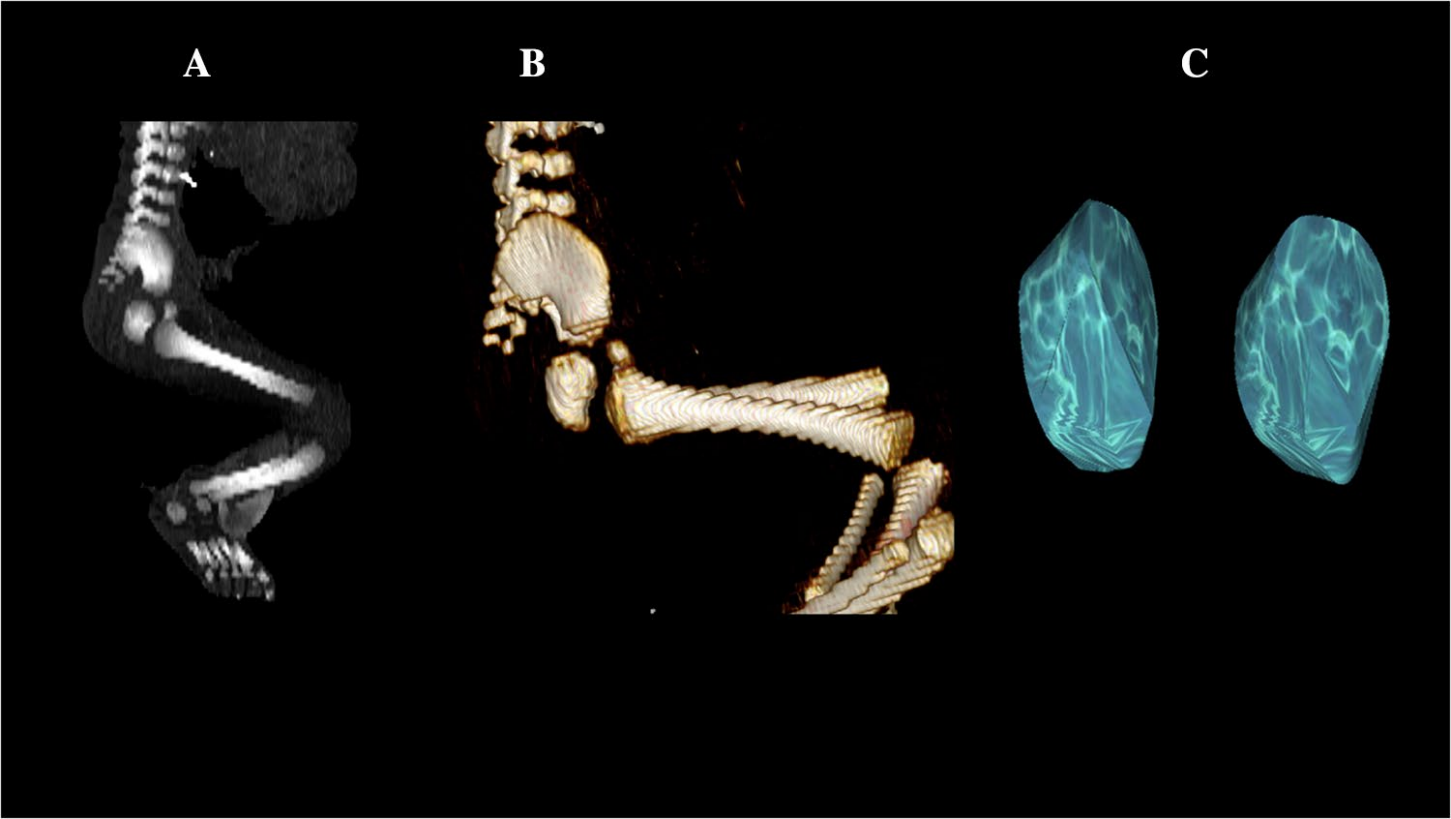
CT of a male human fetus aged 26 weeks in the sagittal projection (a), 3D reconstruction of the pelvis in the sagittal projection (b), and primary ossification center of the ischium (c) using Osirix 3.9
